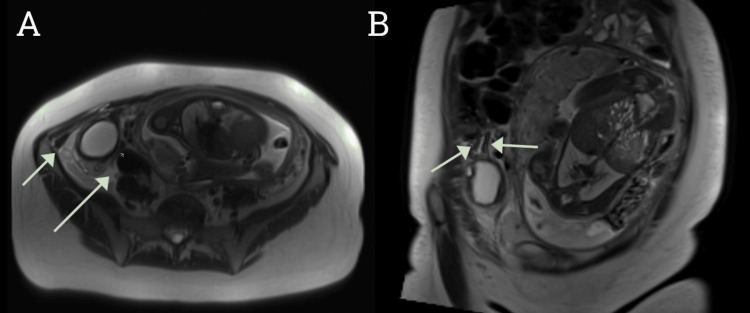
(A) Axial and (B) coronal MRI abdominal images demonstrating inflammatory change adjacent to a cystic structure near the right ovary and thickened tubular structure adjacent to the cecal pole. The arrows show areas demonstrating inflammatory changes. MRI: magnetic resonance imaging
